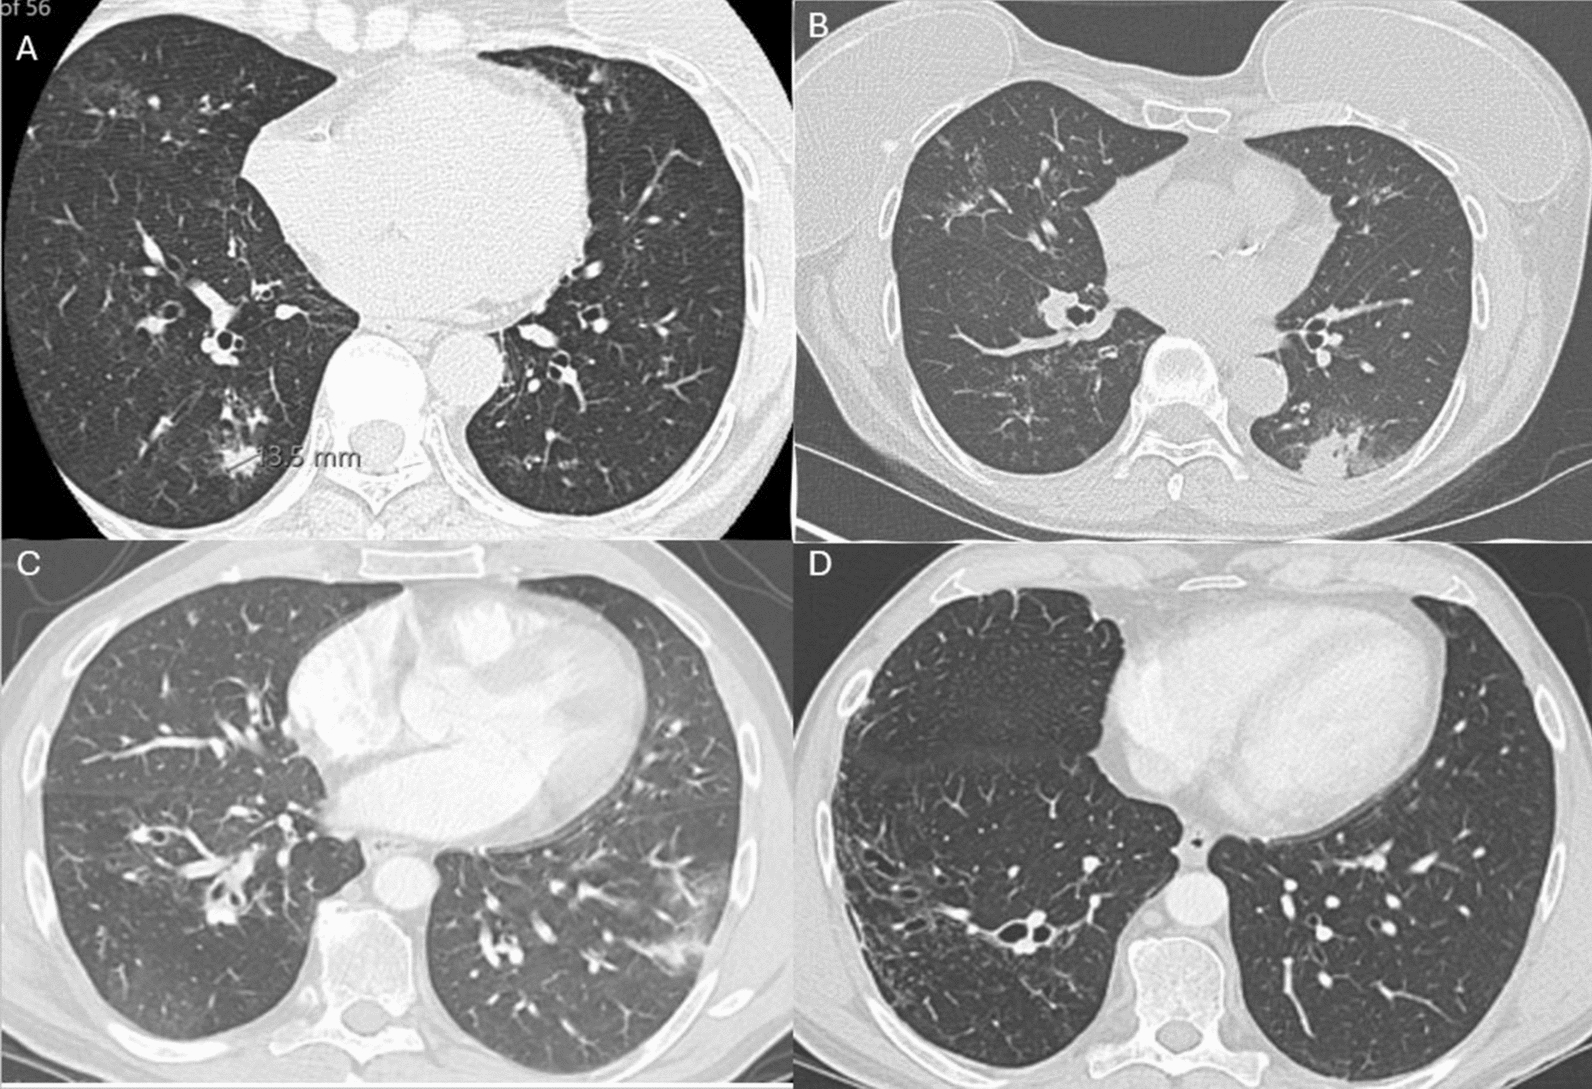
Axial chest CT images before and after dual-biologic therapy with omalizumab + mepolizumab. A. Patient 1 Pre-duo therapy scan reveals bilateral bronchiectasis with dense mucus impaction and patchy consolidation, most pronounced in the right lower lobe. B. Patient 1 follow-up scan after 6 months of duo biologic therapy shows right sided but stable mucus impaction with no progression of bronchiectasis. C. Patient 2 Pre-duo therapy scan shows central cylindrical bronchiectasis, mucus plugging, and mild mosaic attenuation. D. Patient 2 follow-up scan after 43 months of duo biologic therapy demonstrates unchanged bronchiectasis without new parenchymal injury or additional mucus burden, indicating radiographic stability despite corticosteroid withdrawal
